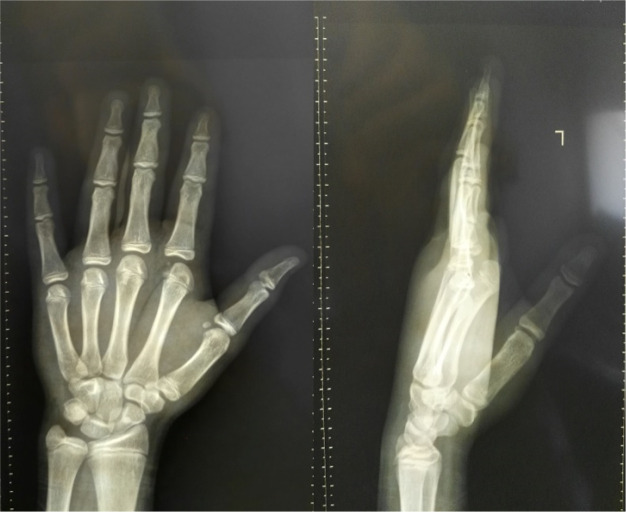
Left hand anteroposterior (left) and lateral (right) radiographs preoperatively demonstrate index metacarpophalangeal joint space widening, index metacarpal epiphyseal head fracture, and dorsal-ulnar displacement of the osteochondral fragment
